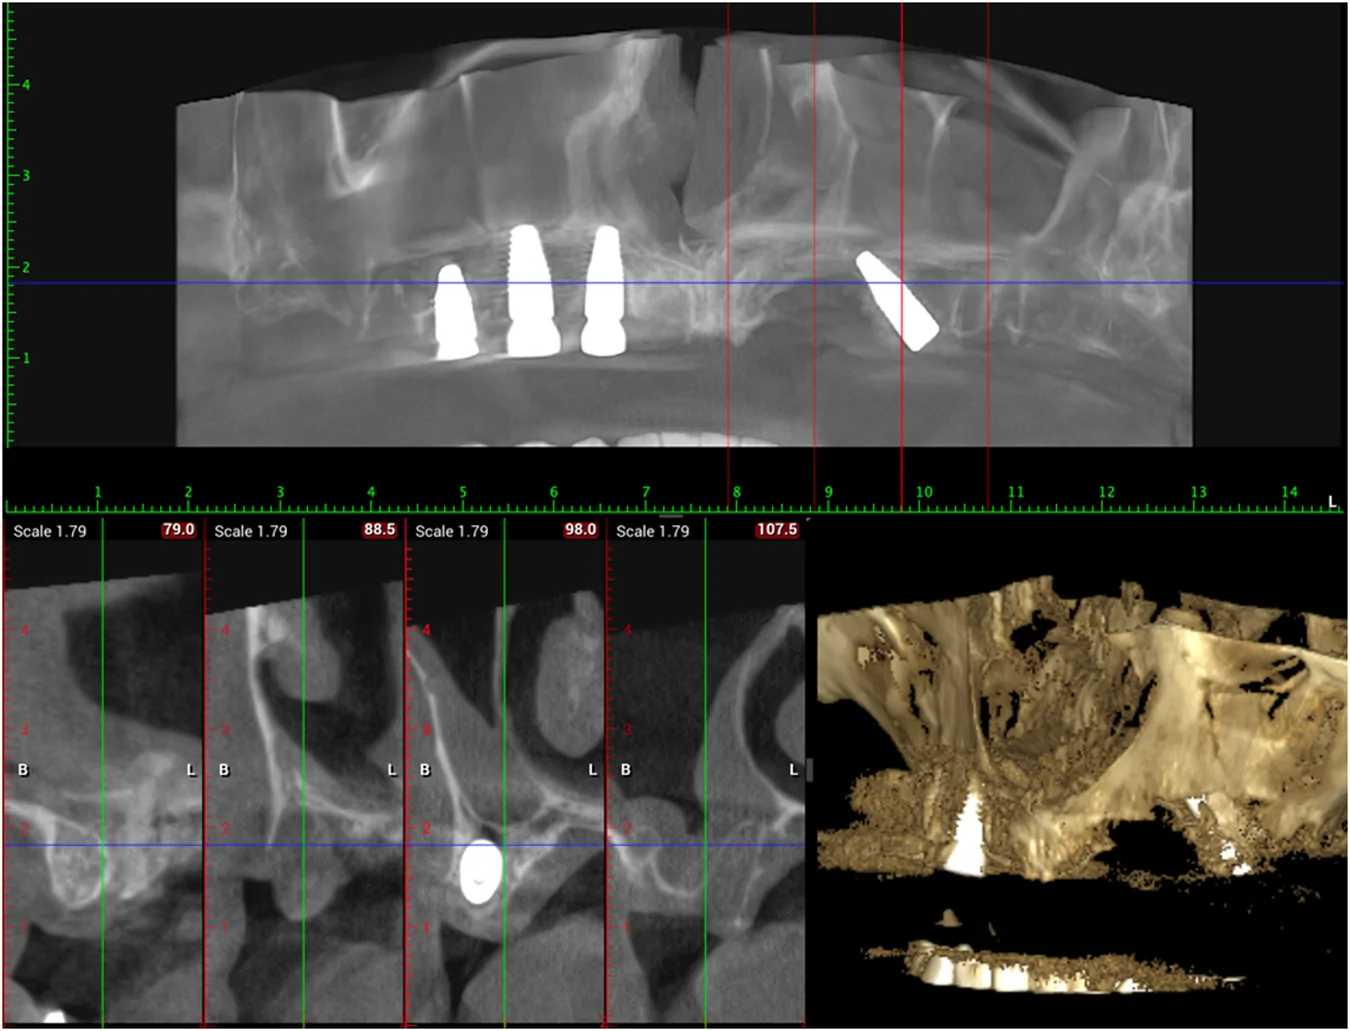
Case 2 Pre-op. Pre-op CBCT shows a 3 cm region with significant loss in bone height in the reconstructed panoramic radiograph (top) and loss of bone width in the sagittal slices (bottom left) and 3D reconstruction (bottom right).
